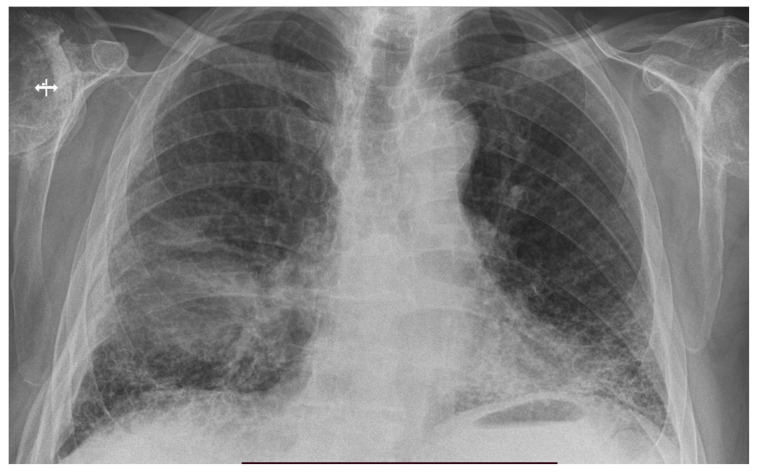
Chest X-ray showing right lower lobe opacity and fibrosis.
